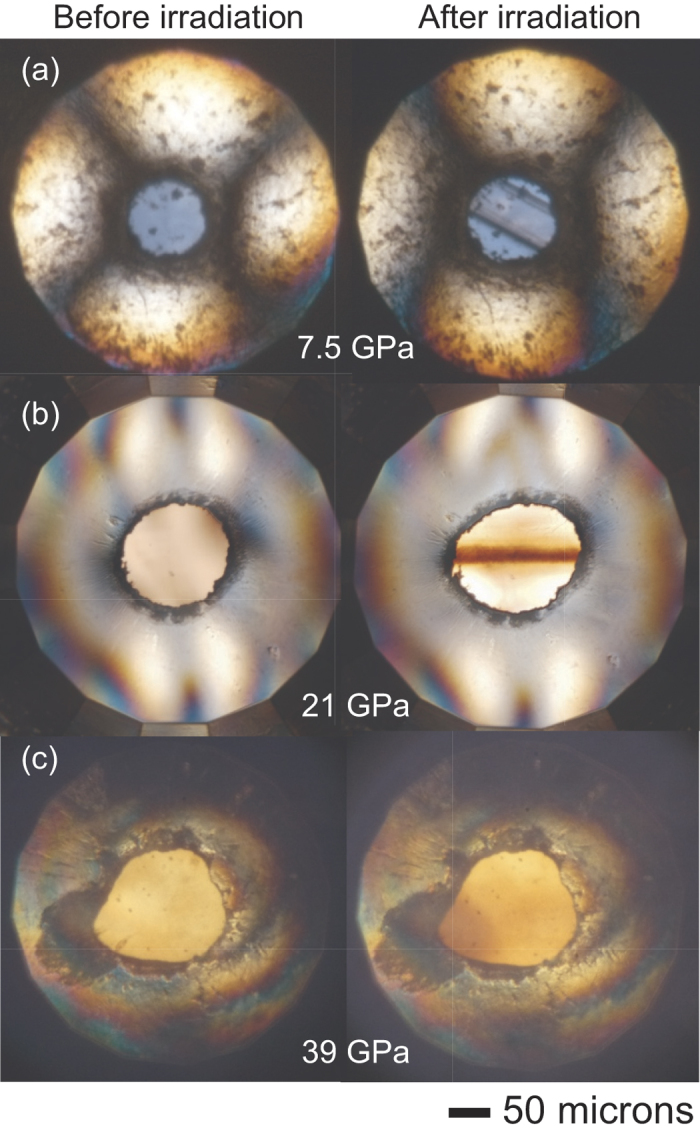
Microphotographs of H_2_O in diamond anvil cells with reflected and transmitted illumination before (left panels) and after (right panels) 10-keV X-ray irradiation at (a) 7.5, (b) 21, and (c) 39 GPa pressures. The X-ray is incident from the upper left in (a), the left (b), and the lower left in (c). The color where the incident X-ray passed changed at 7.5 and 21 GPa whereas there was almost no change observed at 39 GPa. The scale bar is 50 μm.
